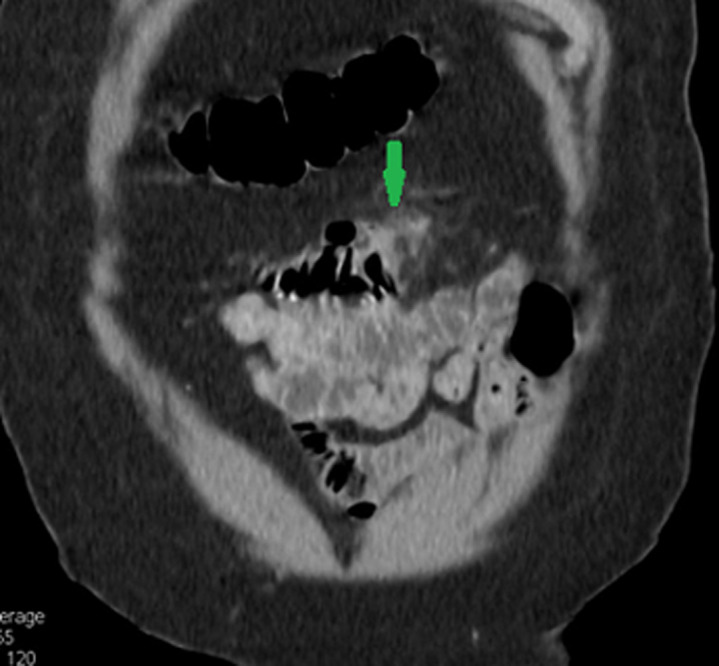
CT-scan (coronal view) showing a surrounding significant inflammatory reaction
